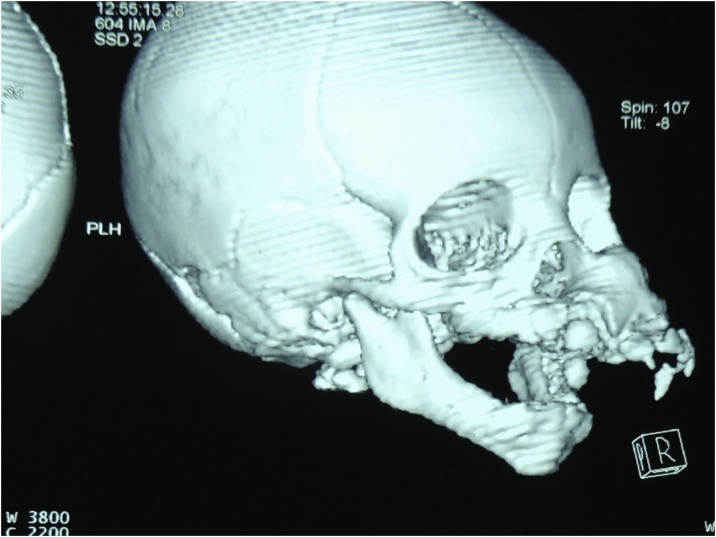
Preoperative 3D reconstruction CT of patient 2, showed marked expansion of the Left maxilla.
